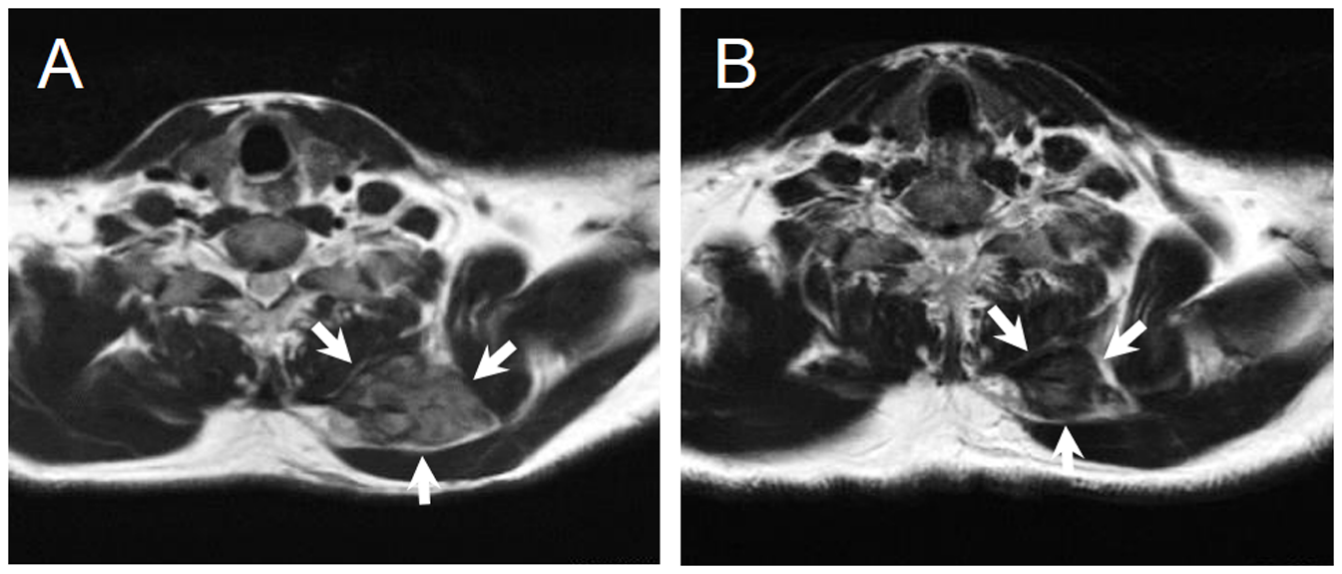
Forty-four year-old man with a desmoid tumor arising in the left posterior neck region. Evaluation for efficacy of meloxicam according to RECIST criteria was PR. This tumor had T41A mutation. (A) A T2-weighed axial MRI of desmoid (arrows) at the first visit. (B)A T2-weighed axial MRI of desmoid (arrows) at last follow-up.
